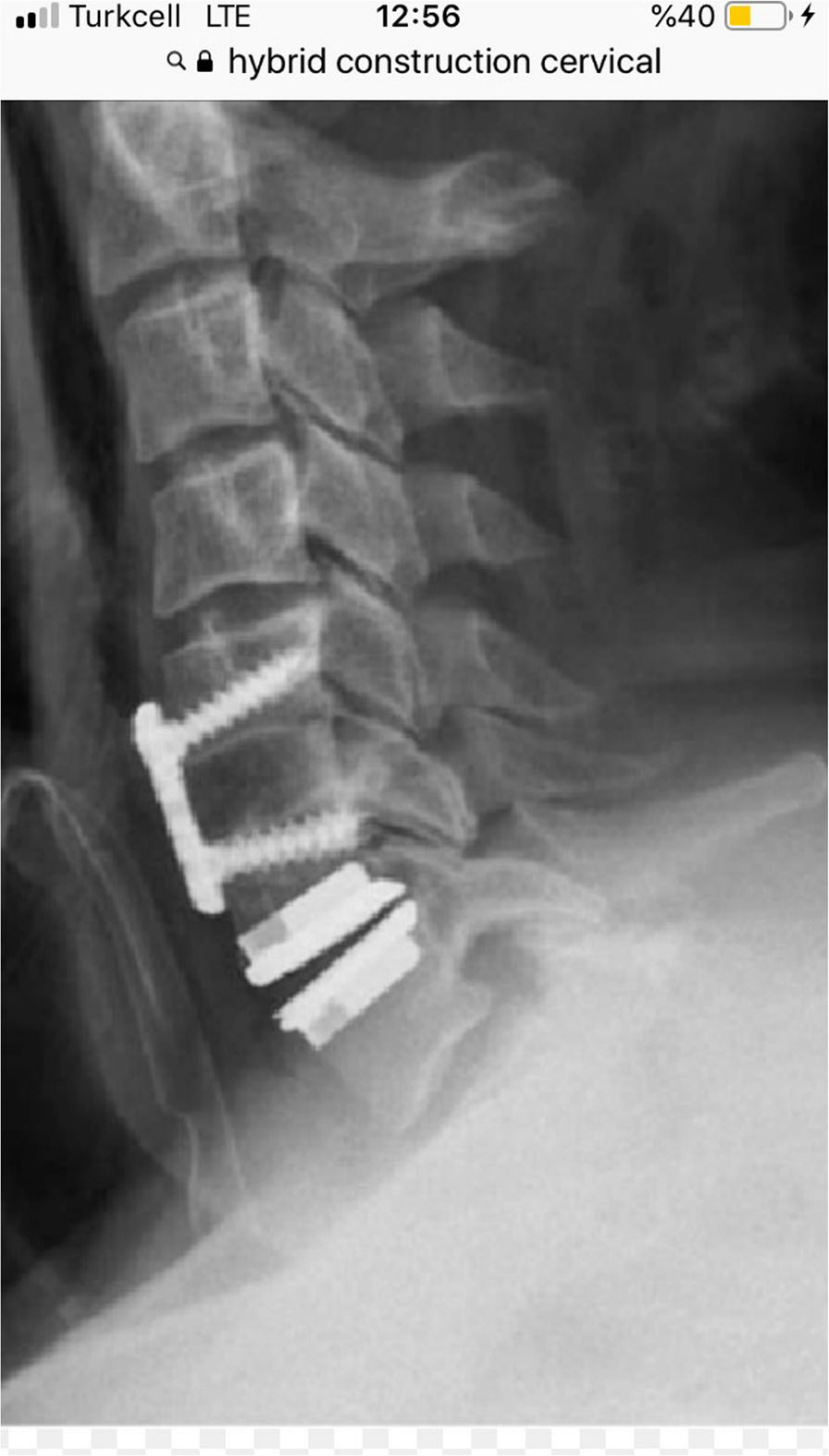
Plain radiograph demonstrating hybrid construction with anterior fusion for C5–C6 and cervical disc prosthesis for C6–C7
